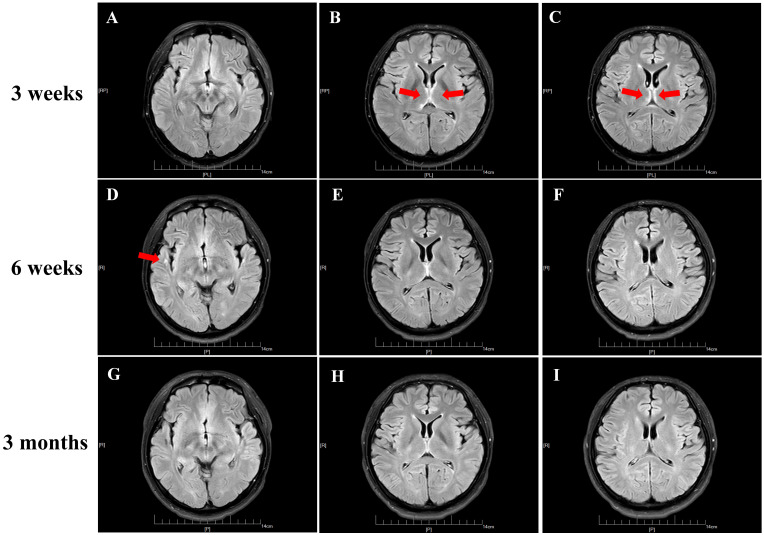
(A–C) Brain MRI of the patient at three weeks after the symptom onset showed hyperintensity of the extensive cortex in bilateral thalamus on fluid attenuated inversion recovery (FLAIR) imaging. (D–F) MRI was repeated at six weeks and showed a significant improvement of the imaging abnormality hyperintensity in bilateral thalamus, but hyperintensity in Right temporal lobe. (G–I) MRI performed at almost three months after initial symptom onset depicted no signal abnormalities on FLAIR sequences.
